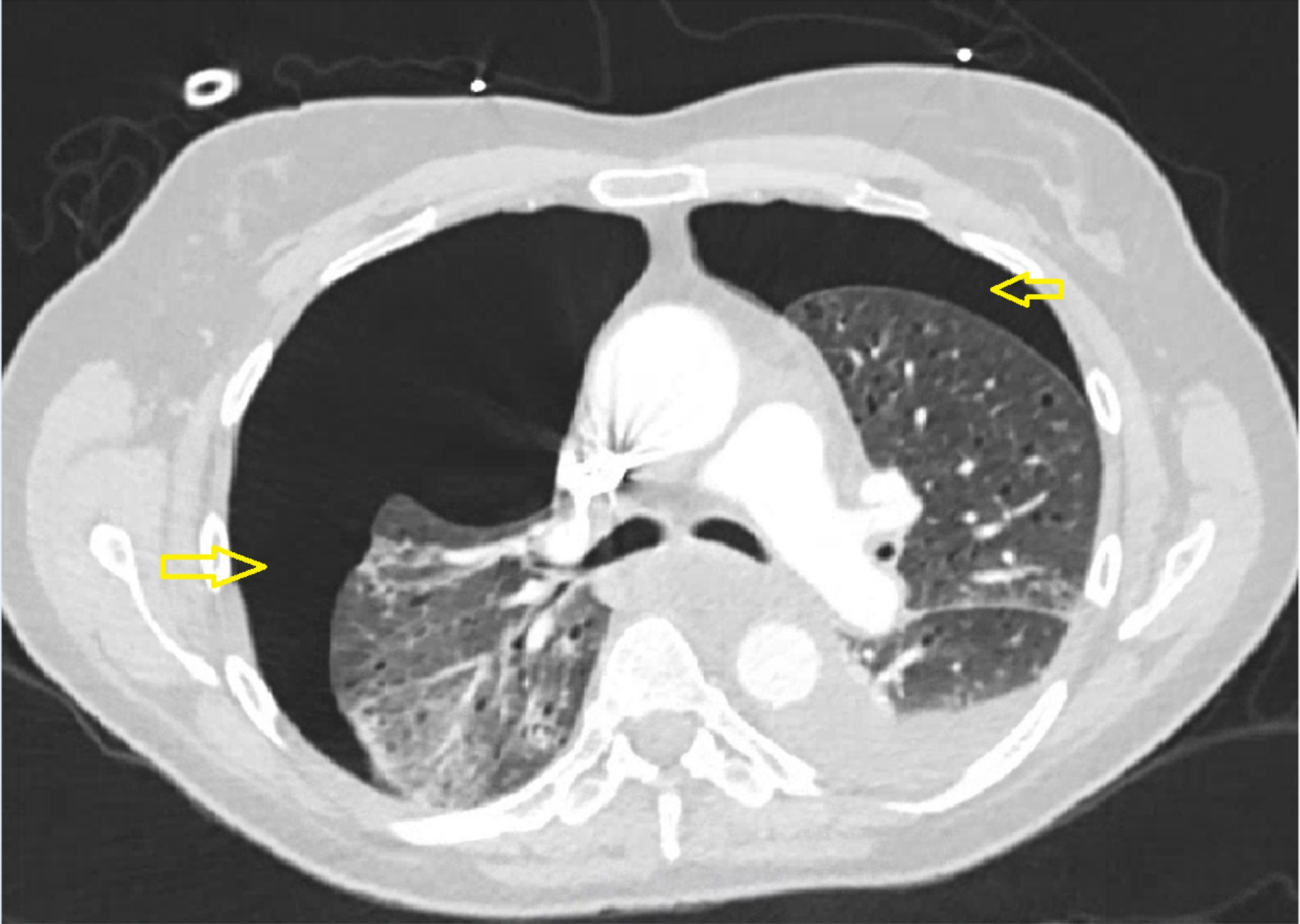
CT angiography of the chest showing bilateral pneumothoraces (yellow arrowhead); large right pneumothorax and moderate left pneumothorax with a left pleural effusion and diffuse bilateral cystic lung disease.
